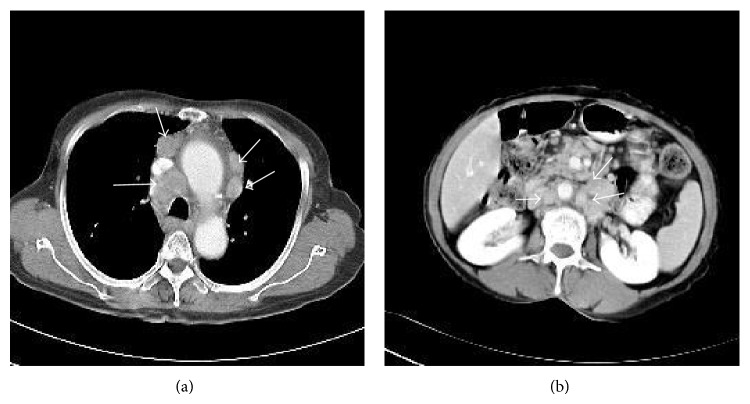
(a) A chest CT shows multiple enlarged lymph nodes in mediastinum. (b) An AP CT reveals multiple enlarged lymph nodes in abdominal retroperitoneal space. (White arrows: enlarged lymph nodes.)
